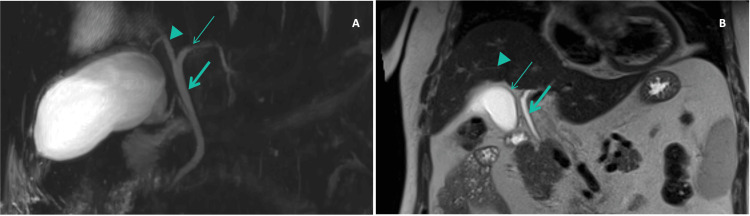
Magnetic resonance cholangiopancreatography (MRCP) performed during the first admission, a few days after the abdominopelvic CT (A) Coronal T2-weighted HASTE (half-Fourier acquisition single-shot turbo spin-echo) MRCP image showing no dilatation of the main bile ducts, with visualization of the common hepatic duct (thick arrow), left hepatic duct (thin arrow), and right hepatic duct (arrowhead). (B) Heavily T2-weighted cholangiographic MRCP image demonstrating no dilatation of the extrahepatic bile ducts (thick arrow) or intrahepatic bile ducts (arrowhead). There is also marked improvement in the gallbladder wall thickening (thin arrow) previously seen on CT, despite persistent cholestasis.
